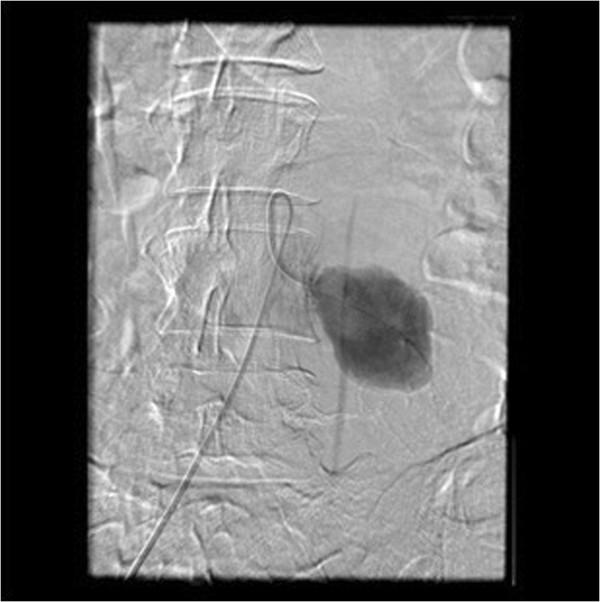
CT Angiogram: metallic device from the embolisation of the pseudoaneurysm of the left lumbar artery.
